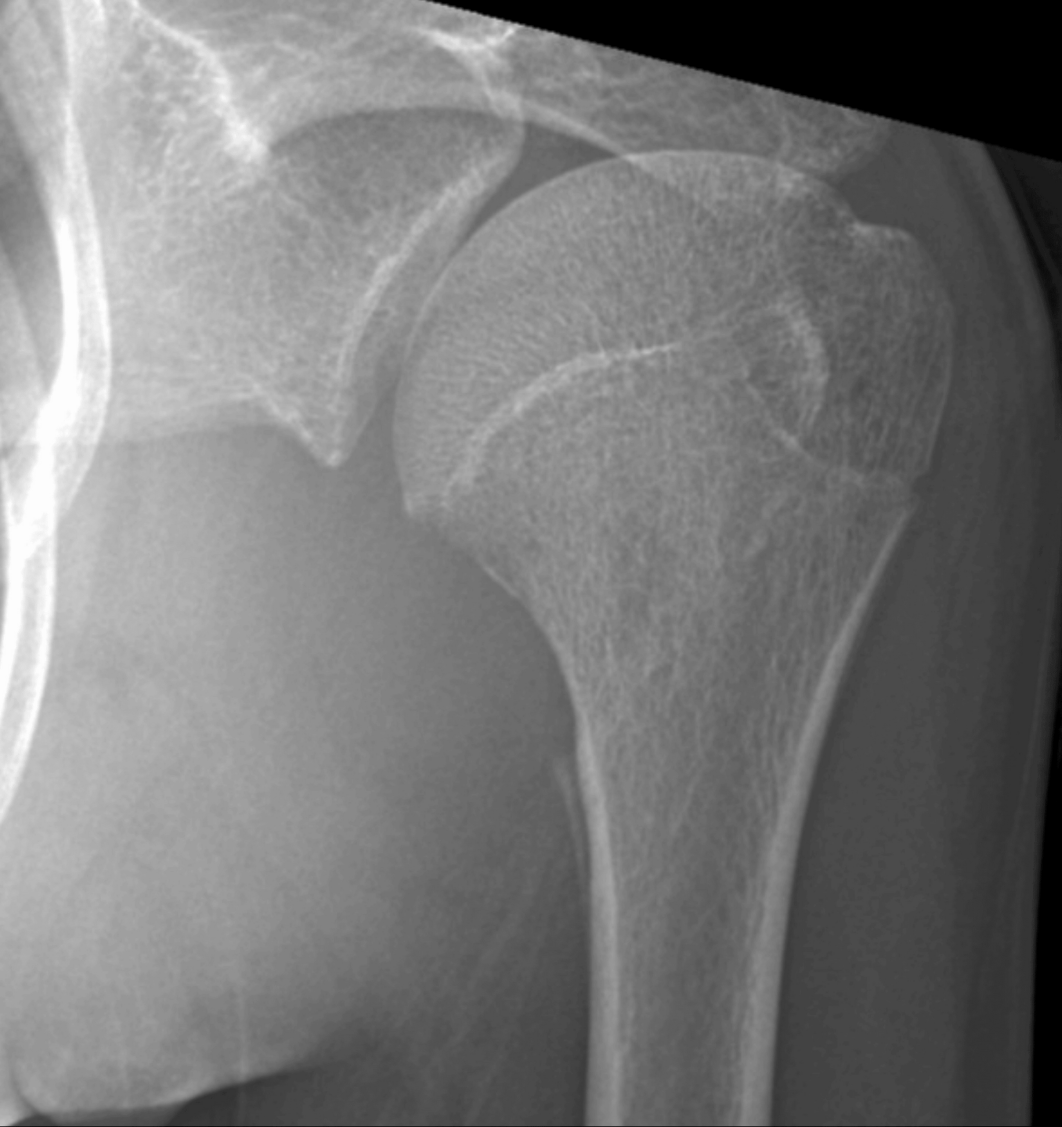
Frontal radiograph of the left shoulder Subtle periosteal reaction is seen along the medial aspect of proximal humerus. Also noted is a small area of ill-circumscribed erosion along the inferomedial aspect of humeral head.
